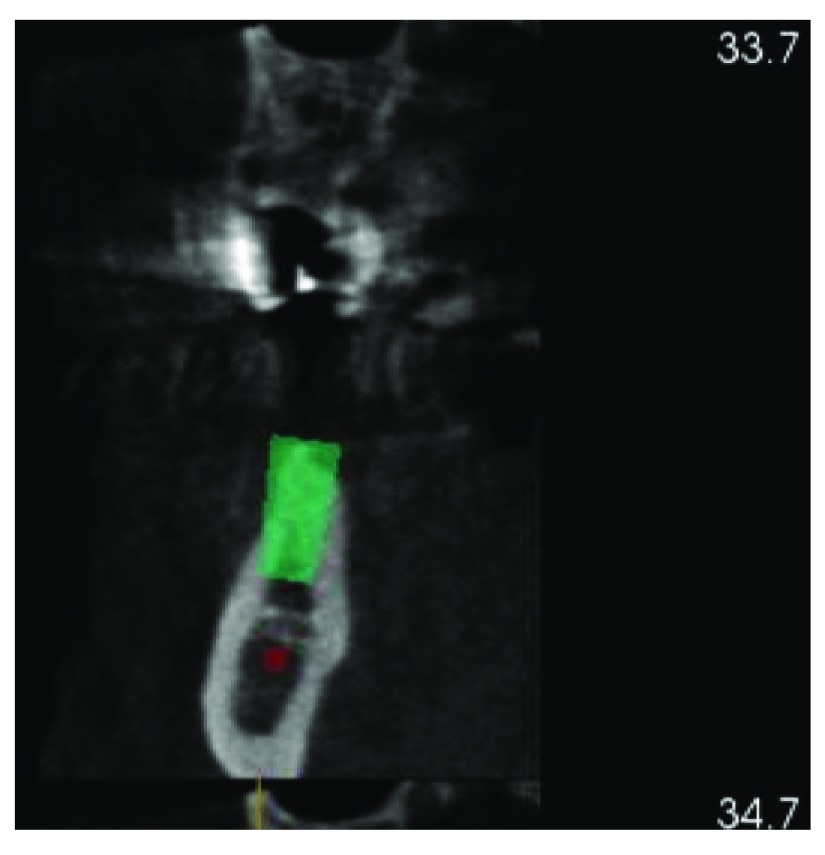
CBCT showing insufficient bone width at tooth #46.
